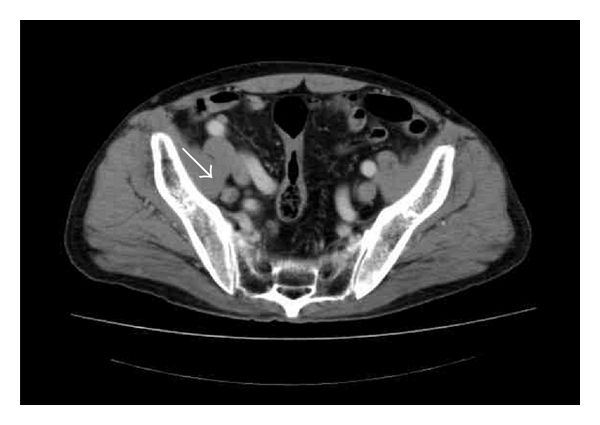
Computed tomography revealing metastases in pelvic lymph nodes.
